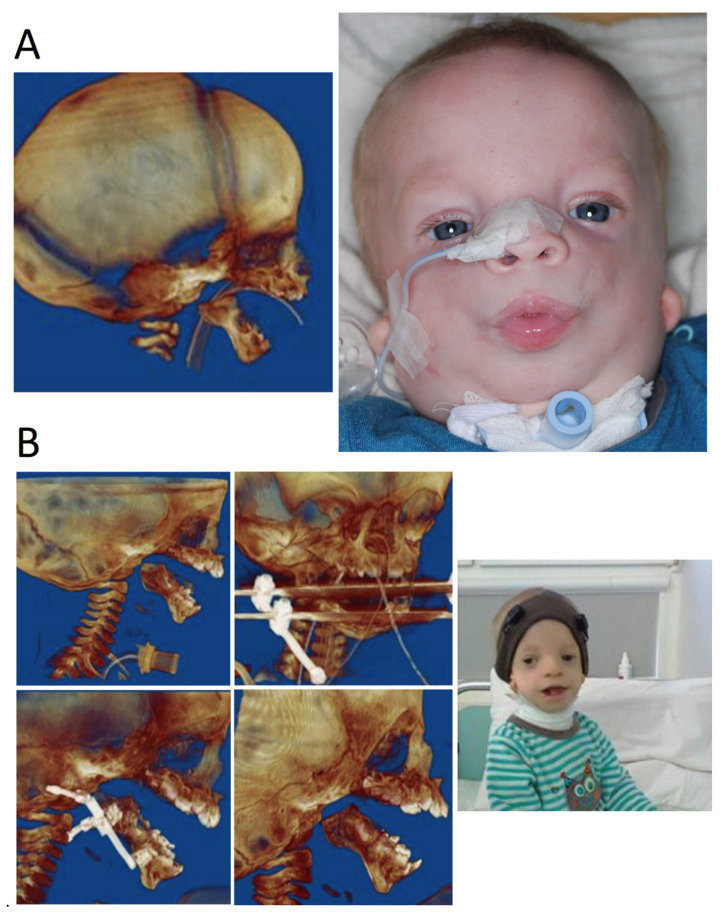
The same patient at different ages during different stages of treatment. (A) Infant with TCS, CT image of 3D reconstruction, patient with tracheostomy. (B) Few years-old patient. 3D images of reconstruction during treatment—multistage mandibular osteodistraction; patient after completion of osteodistraction process, removed tracheostomy tube.
